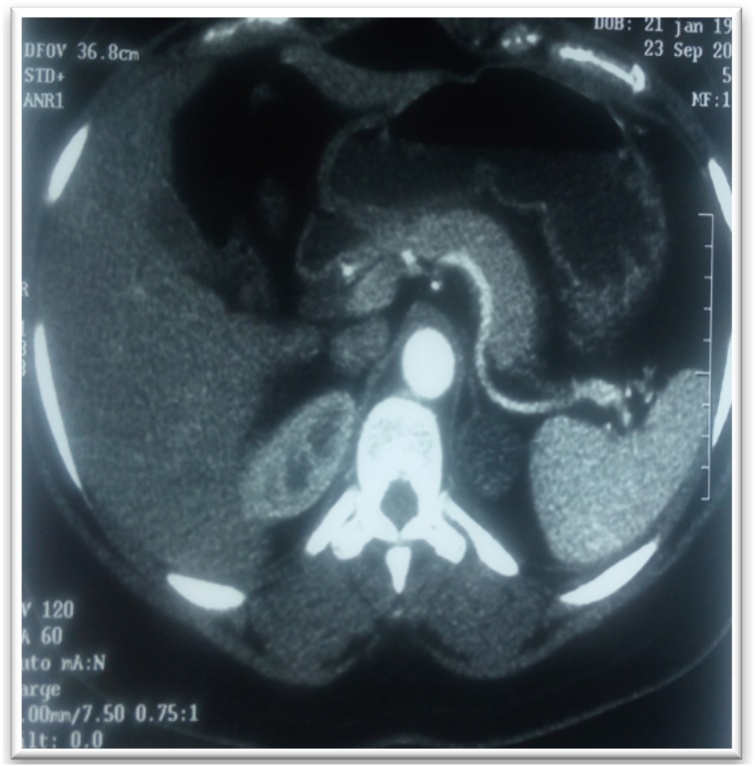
Abdominal CT showing a left adrenal mass (case 6).
